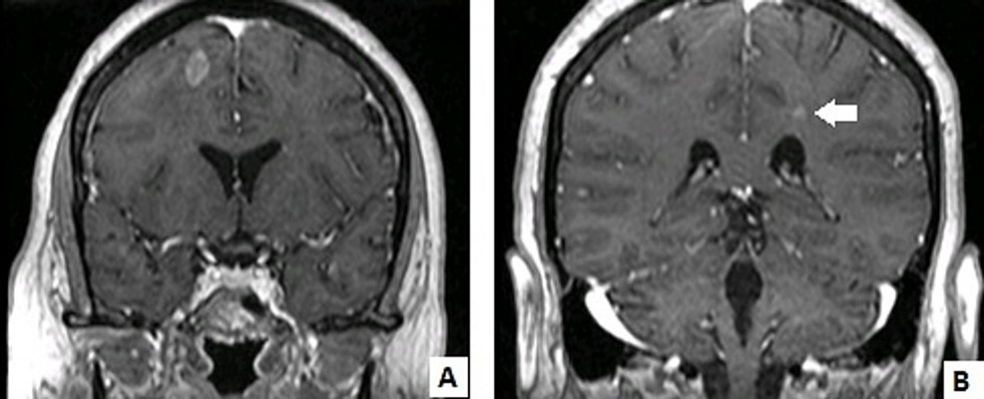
Cranial Magnetic Resonance imaging after two cycles of TDM-1. A: Right frontal lobe lesion with 9x8 mm.  B/Arrow: Left front parietal lobe lesion with 4 mm.
